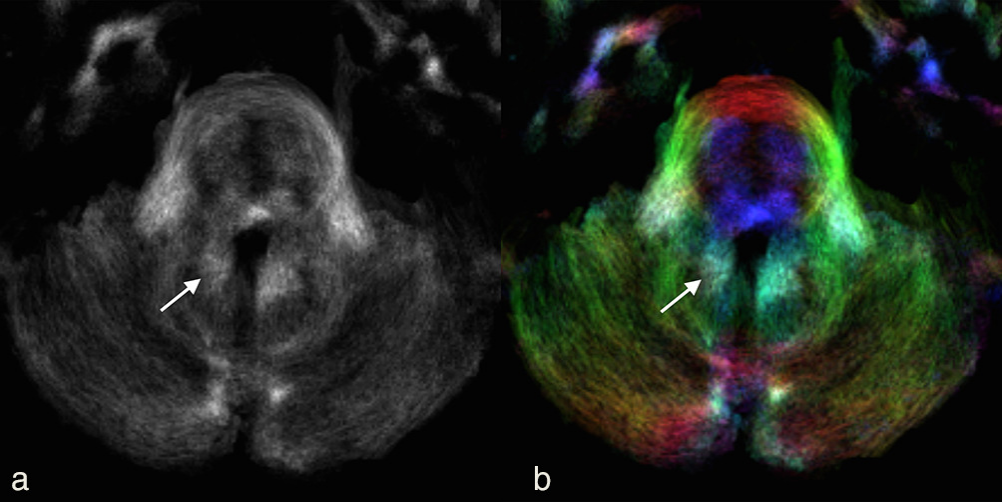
(a) Axial track density imaging and (b) direction-encoded colour-track density imaging at the level of the pons (same level as Figure 1a) 3 months after the initial MRI showing asymmetric probabilistic streamline loss in the right dentate nucleus and denato–rubral track (arrows) compared with the contralateral side.
